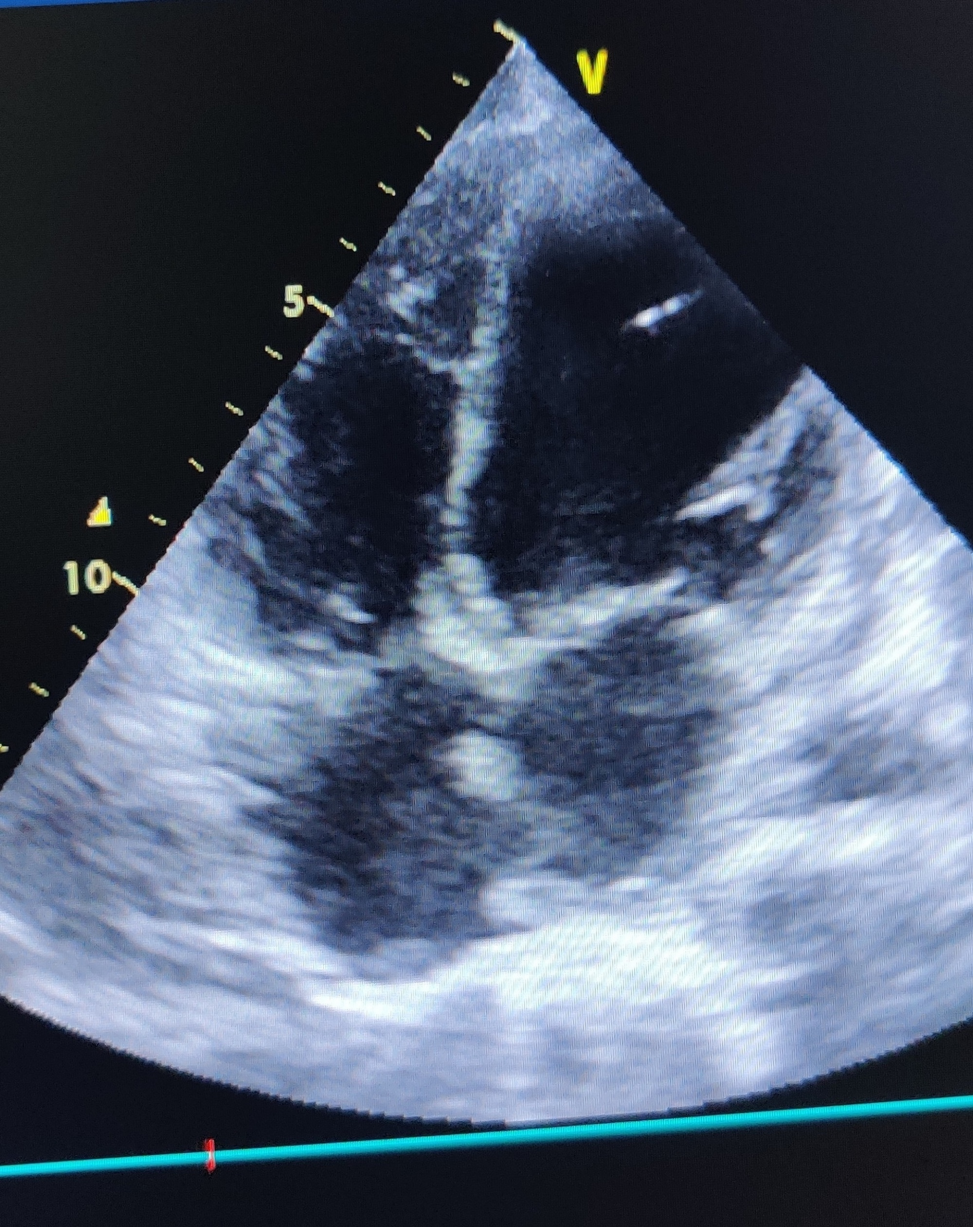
Repeat 2D echocardiogram (ECHO) in the present case on day 7
